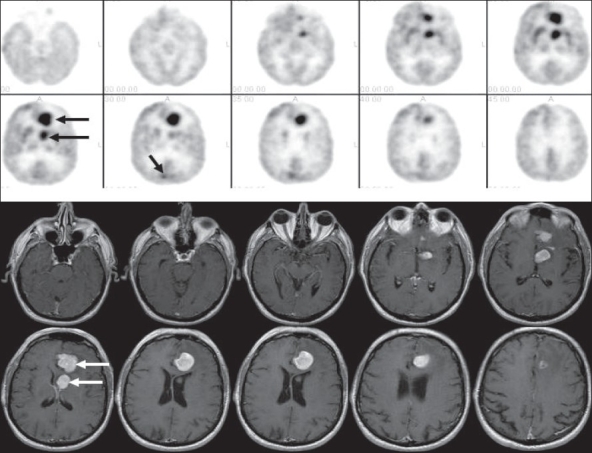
Axial brain images with FDG-PET (top two rows) and MRI (bottom two rows) in a patient with CNS lymphoma. Although it is difficult to assess malignant lesions in the brain, CNS lymphoma lesions are usually very intense on FDG-PET imaging; and as shown in this example, sometimes more lesions can be seen with FDG-PET brain imaging as compared to brain MRI (arrows)
